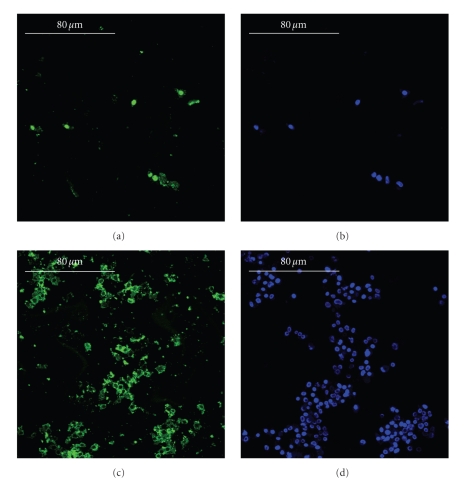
Immunolocalization of type-I collagen (panels a and c, green) and cellular nuclei (panels b and d, blue) in the static culture (panels a and b) and in the ultrasonic culture (panels c and d), bars equal to 80 *μ*m. During the culture period, in the control (panels a and b), the osteoblasts built a scanty amount of bone matrix, whereas, in the stimulated culture (panels c and d), the osteoblasts secreted a wide amount of matrix. The immunolocalization of osteocalcin, osteopontin, and type-III collagen revealed similar results.
